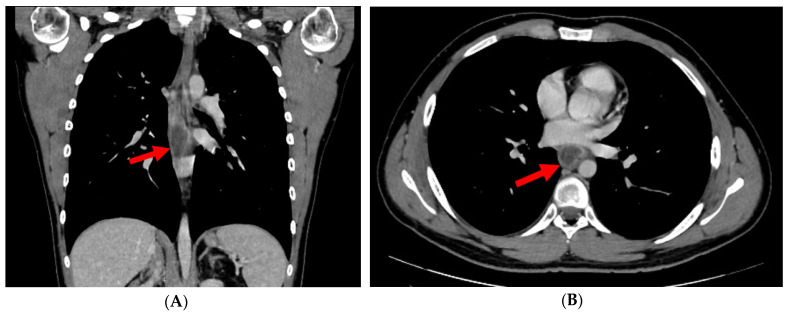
(A,B) Contrast-enhanced CT scan performed during the venous phase of contrast circulation, in axial (A) and coronal planes (B): Localized intramural collection located in the right anterolateral aspect of the middle thoracic esophagus, with modified fluid densities (34 Hounsfield Units) and slight mass effect on the esophageal lumen, which is displaced medially (red arrows).
